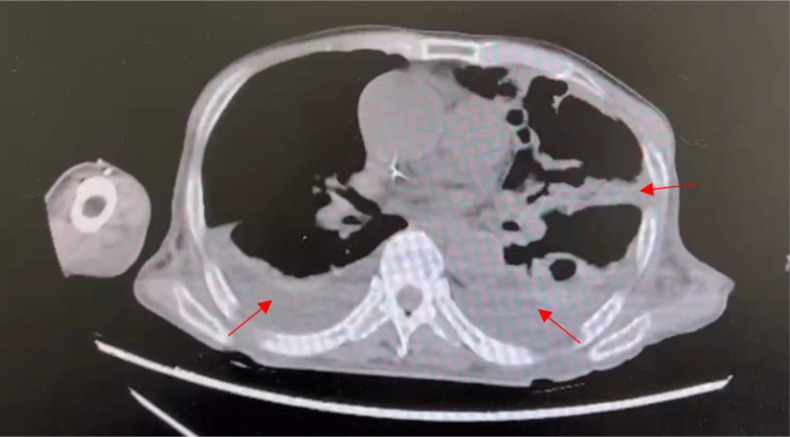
Transverse section from chest CT scan of patient 1 showing pleural effusion, hyperdense regions surrounding the lungs, as indicated by the arrows. Pleural effusion is characteristic of pulmonary infection.
